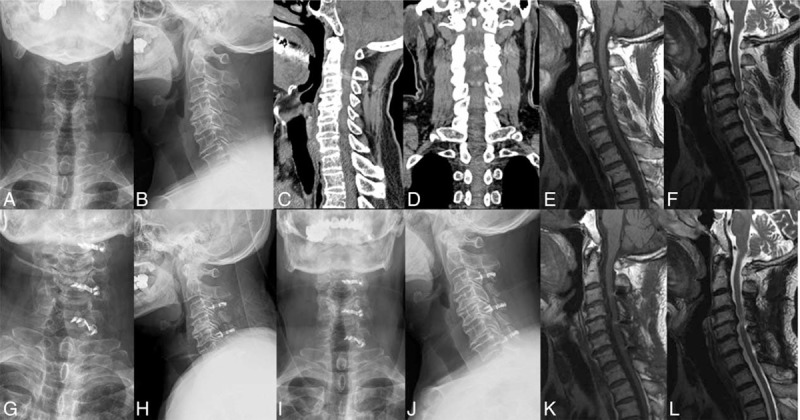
CSM: A 63-year-old male patient (A-L), preoperative X-ray (A, B), CT (C, D), MRI (E, F), 1 week (G, H), and 1 year (I, J) after operation the X-ray showed good internal fixation; 1 year after operation the MRI examination (K, L) showed spinal cord without compression. CSM = cervical spondylotic myelopathy.
